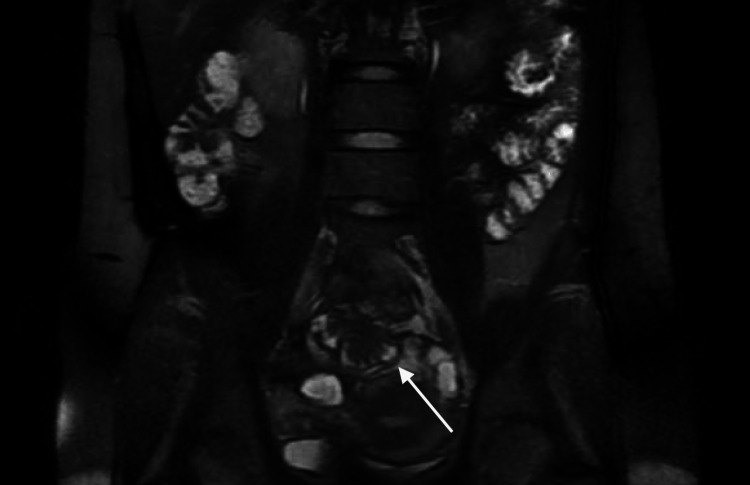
MR enterography with coronal T2-weighted image demonstrating infiltrating mass-like tissue (arrow) along a loop of distal small bowel with associated luminal narrowing.
